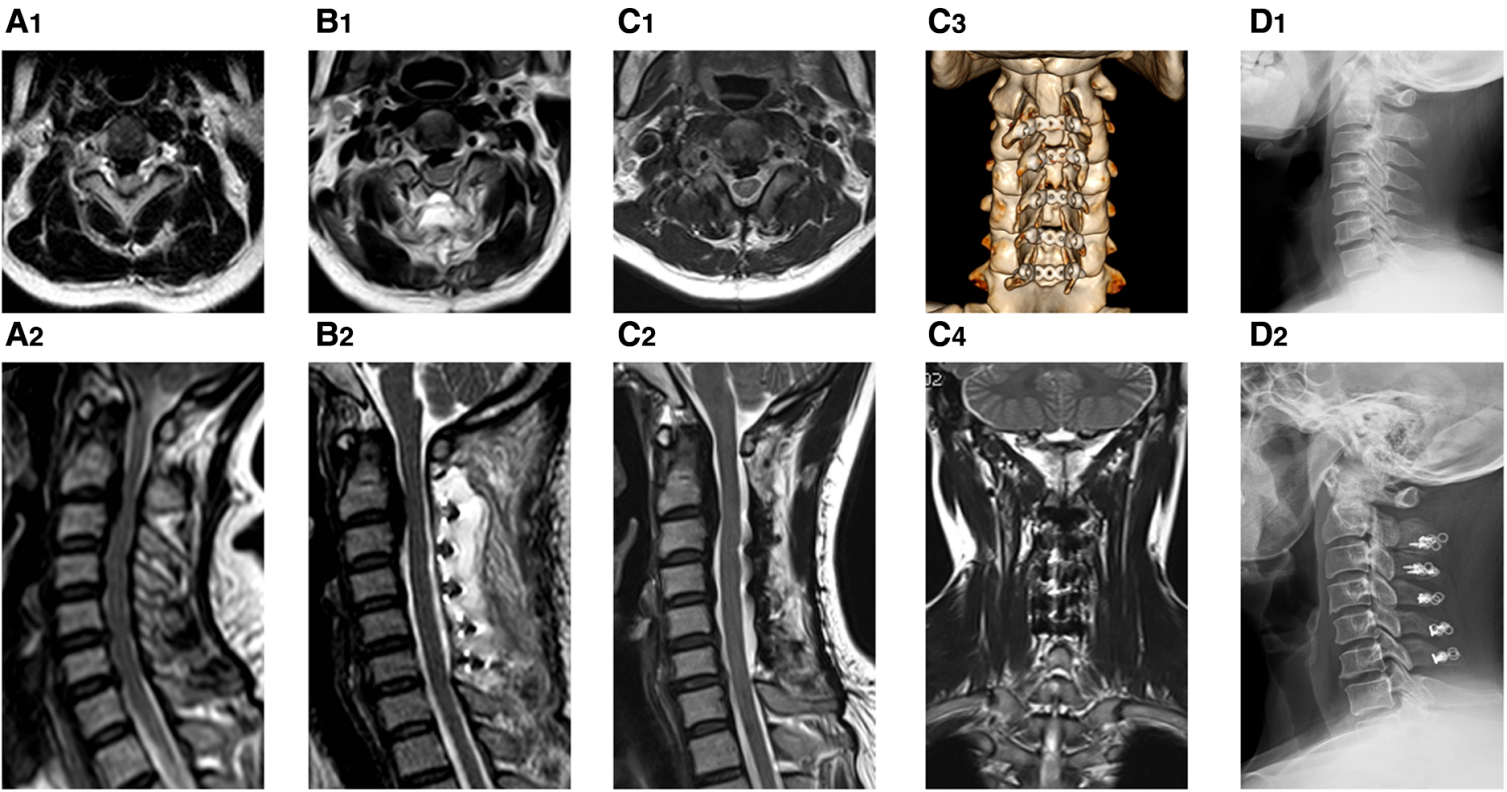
The pre-operative and post-operative images of the procedure. The patient was a 50-year-old female suffering CSM and OPLL. (A1,A2) the preoperative transverse and sagittal T2 images; (B1,B2) the postoperative images at 3 months post operation with transverse and sagittal T2 images; (C1,C2) the postoperative images at 12 months post operation with transverse and sagittal T2 images showing an obvious muscle recovery; (C3,C4) the 3D reconstruction CT images and coronal image of MRI at 12 months post operation; (D1,D2) the preoperative and postoperative standing lateral x-ray films at 12months follow-up.
